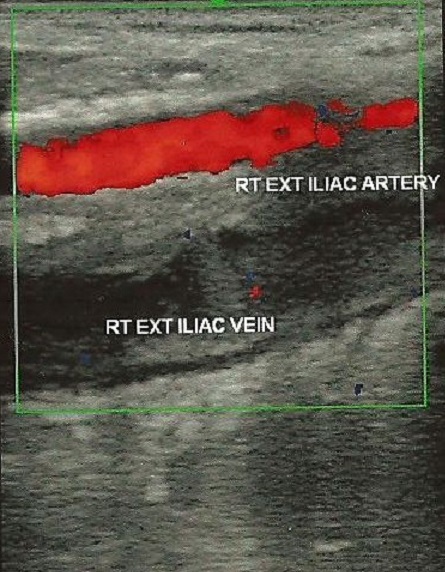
Doppler ultrasound revealed extensive thrombosis involving the iliac veins
